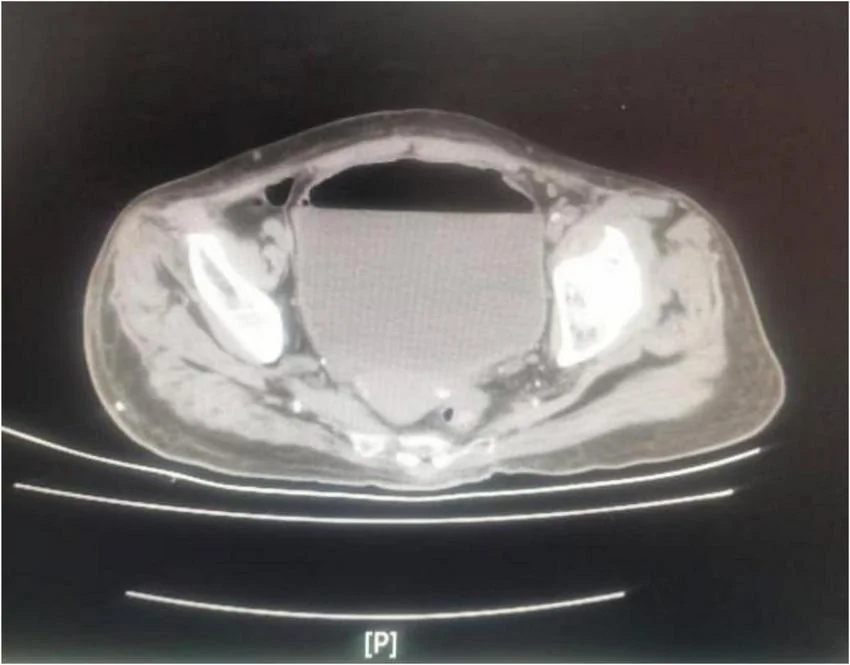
An air-liquid level in the bladder visualized on the abdominal CT scan on April 6, 2025.
